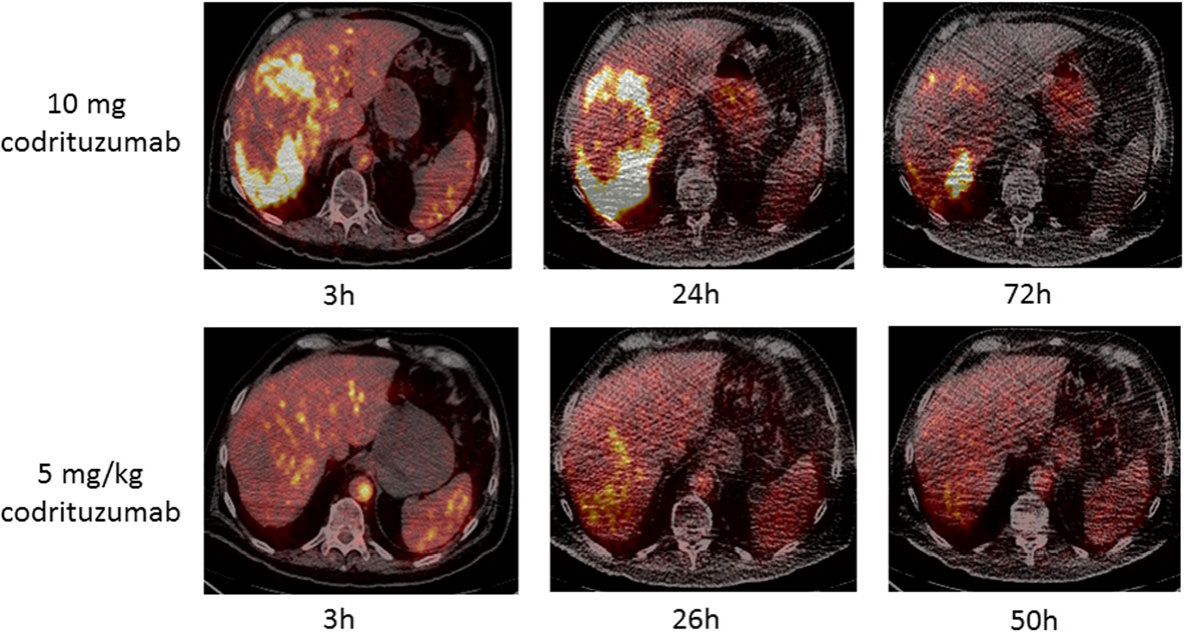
Patient 7, serial-fused I-124 codrituzumab-PET/CT baseline images following injection of 190 MBq of I-124 codrituzumab (10 mg of codrituzumab) (upper panel). Upper panel images show prominent heterogeneous uptake in a large right liver tumor lesion, with more uptakes in the periphery than those in the central region. The lower panel shows serial-fused I-124 codrituzumab-PET/CT images obtained after treatment with sorafenib and codrituzumab (5 mg/kg) for 4 weeks and after injection of 183 MBq I-124 codrituzumab (co-infused with 5 mg/kg of codrituzumab). All images are displayed at the same SUV and show marked decrease in intensity of uptake in the periphery. For example, at 24 to 26 h, the SUVmax decreased in the cold co-infused images (lower panel) from 20 to 40% of the 10 mg injected mass, and in the periphery, there was less of a drop in SUVmax to 80–90% of the 10 mg injected mass, probably because of central necrosis
